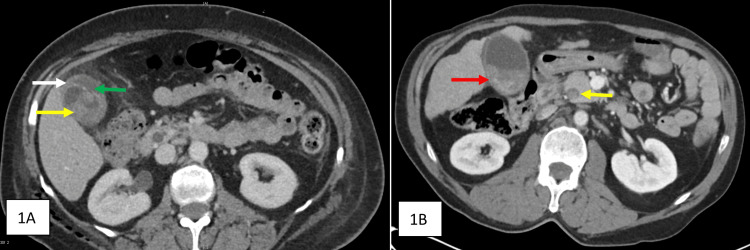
CT scans of the two patients during admission 1A: CT mesenteric angiogram (CTMA) for the female driver at 72 hours post-injury. Hyper-dense material within gallbladder lumen (yellow arrow), discontinuity of gallbladder wall (green arrow) and pericholecystic fluid (white arrow) are seen, which indicate a traumatic perforation with haemobilia. 1B: Initial CT of abdomen and pelvis for the male passenger at the time of presentation. Hyper-density in the dependent portion of the gallbladder lumen (red arrow) and mild pericholecystic fluid can be seen. The CBD is dilated and also contains hyperdense material (yellow arrow).
